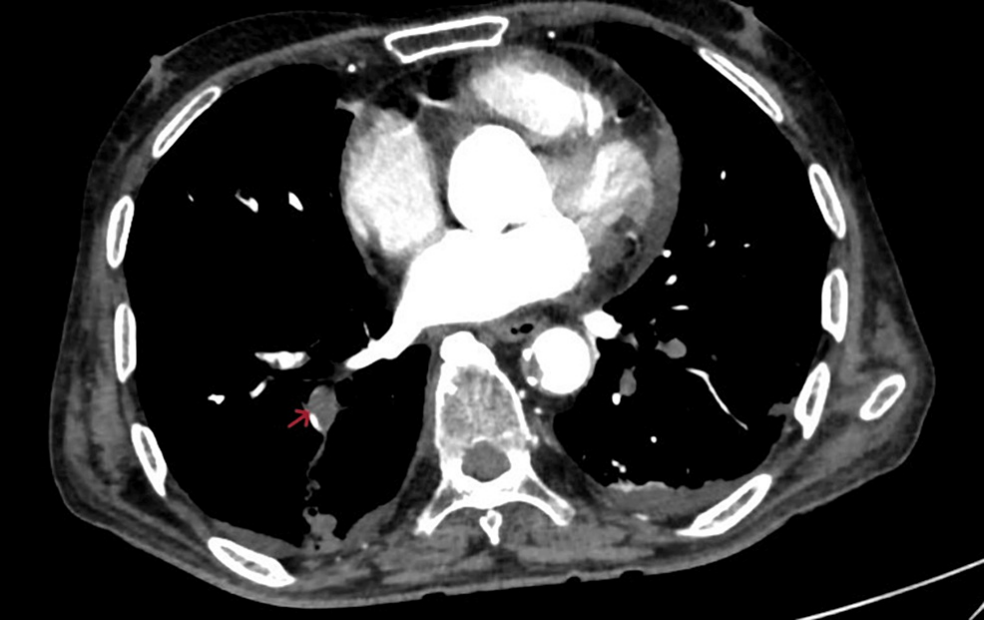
Axial CTPA showing a hypodense filling defect (red arrow) in the lower segmental branch of the right pulmonary artery. CTPA, computed tomography pulmonary angiography
